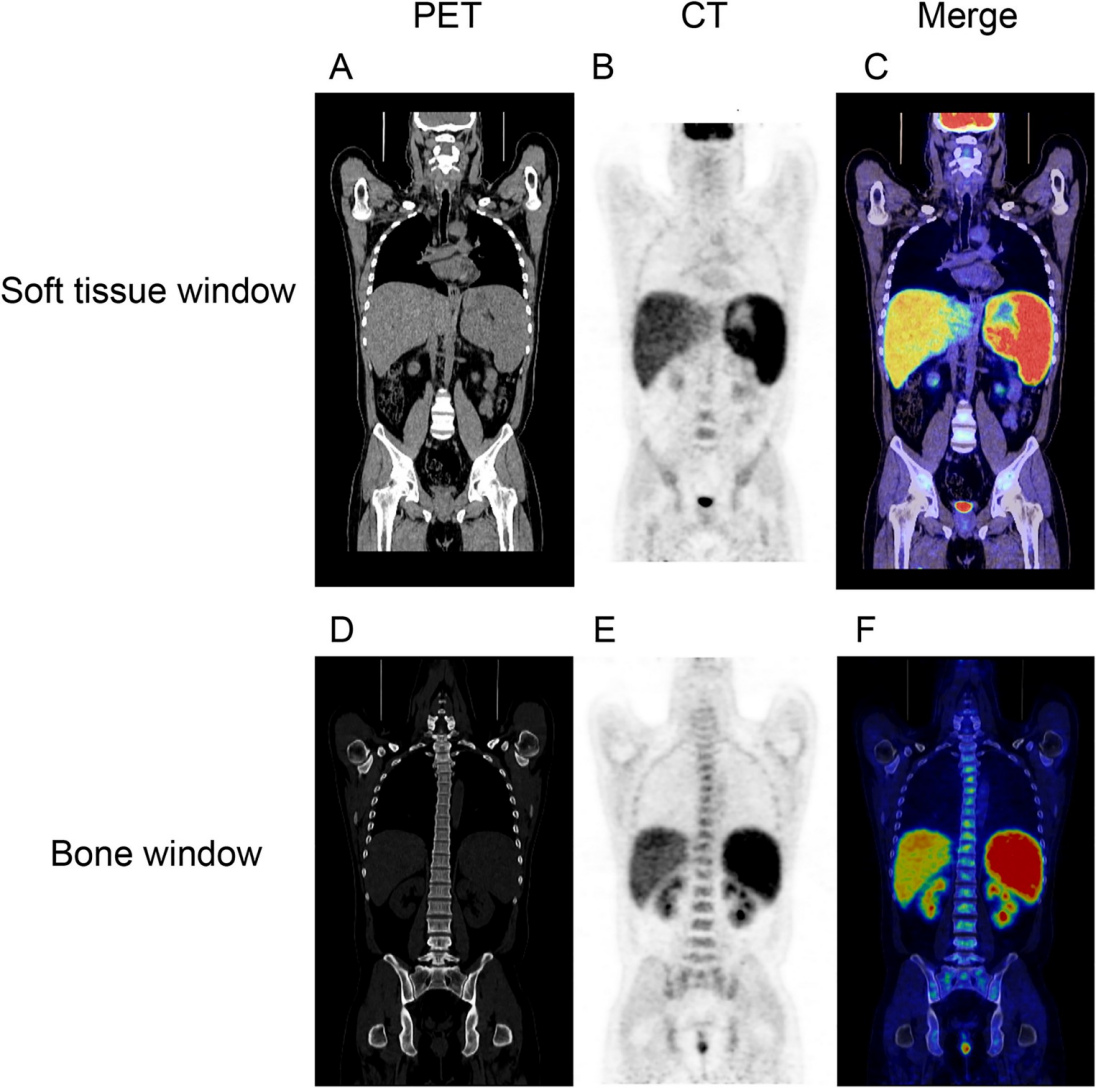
FDG PET/CT scanning in the VL associated HLH case revealed marked hepatosplenomegaly and elevated diffuse uptake in liver. (A) (SUV max = 4.3) (reference range, 2.3 to 3), in spleen (SUV max = 5.6) (reference range ≤2) and in spinal column (SUV max = 2.5) (reference range ≤1) (B, E), the merge image indicated functional disorder without organic lesion (D) in the merge image (C and F).
